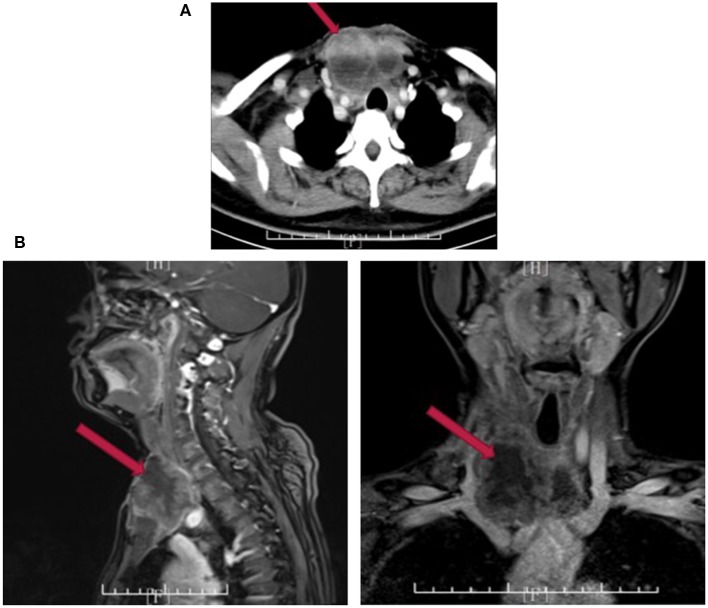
Cervical enhanced CT (A) and MRI (B) 20 days after palliative surgery.
